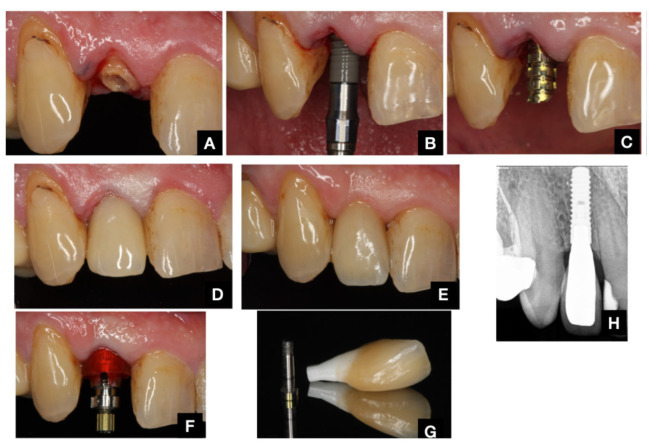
TA protocol. (A): Presurgical situation. (B): Immediate implant placement. (C): Provisional abutment in place. (D): Provisional acrylic restoration in place (T0) (E): Provisional restoration (T = 3). (F): Final impression after 3 months. (G): Final restoration. (H): Final radiograph after 12 months.
